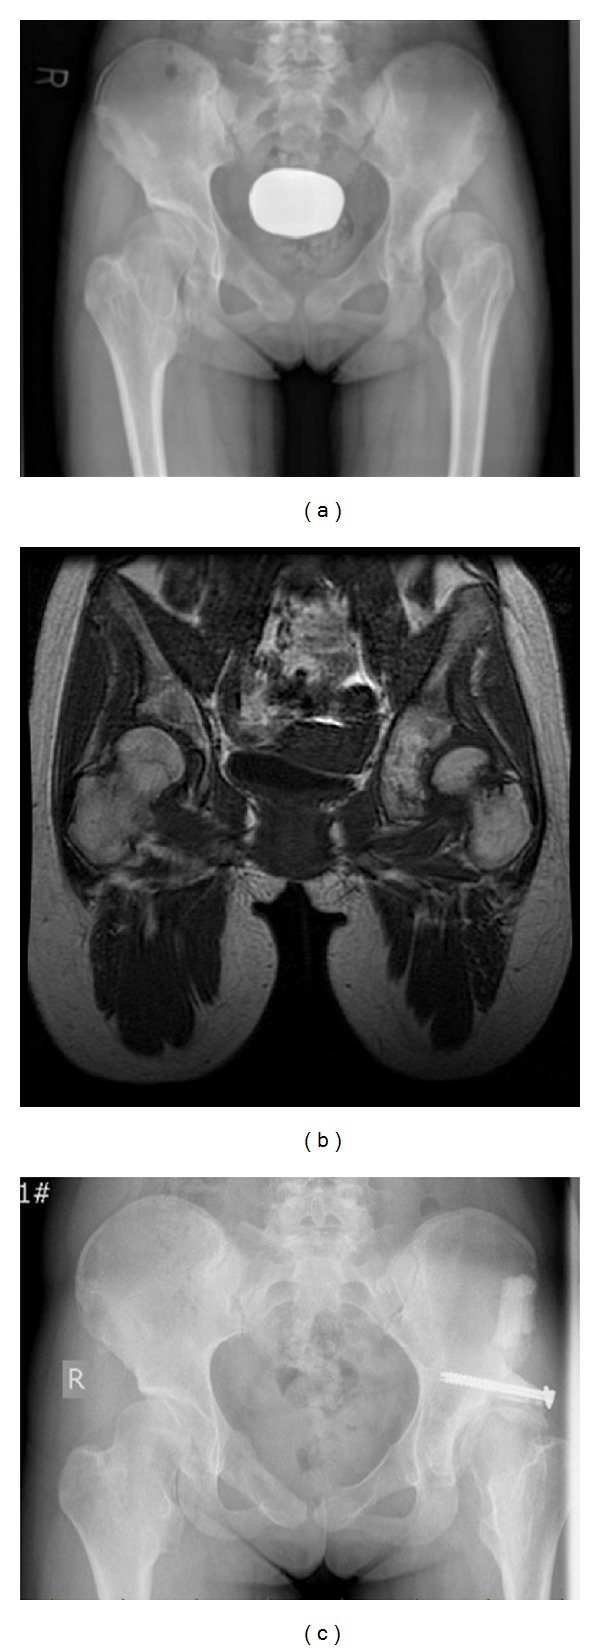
(a) Anteroposterior pelvic radiograph at initial presentation. (b) Magnetic resonance imaging showing a large sessile osteochondroma within the left acetabular fossa. (c) Anteroposterior radiograph of the pelvis 3 years after the left acetabular Shelf procedure and femoral neck osteoplasty.
